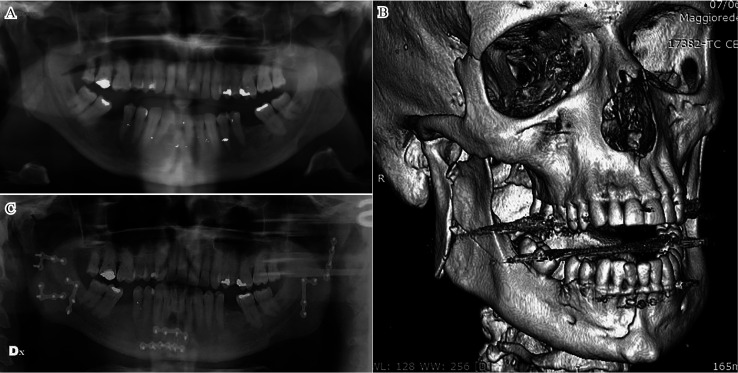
Preoperative panoramic radiograph (A), preoperative 3D CT scans (B), and postoperative panoramic radiograph (C) of a patient with triple mandibular fracture.
